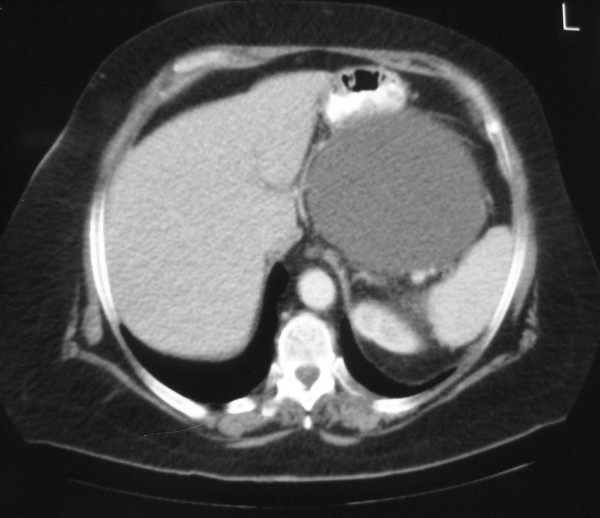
CT axial image after the intravenous administration of a contrast medium demonstrating an intra-peritoneal hypodense non-enhancing mass adjacent to the stomach and spleen.
